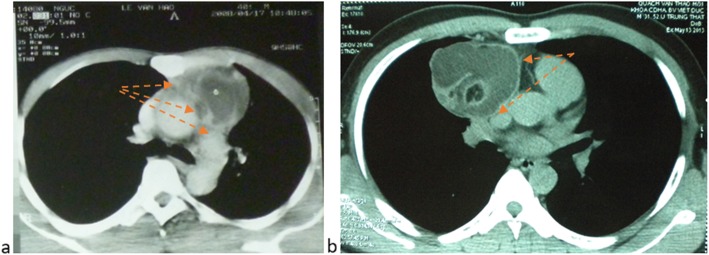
Mediastinal mature teratomas on CT-scanner. a Adhesion lesion with surrounding organs [arrow] – VATS conversion mini-thoracotomy and b clear boundary with surrounding organs [arrow]
